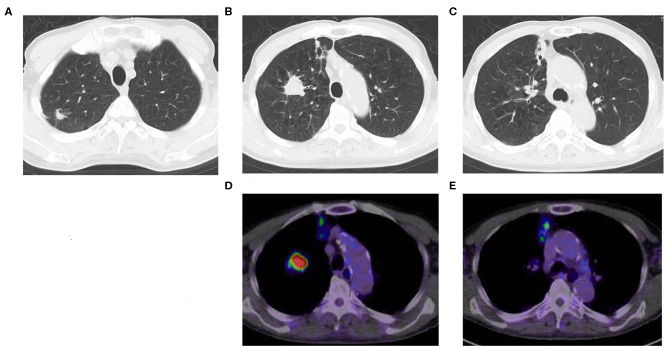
Chest CT shows multiple lung cancers. (A) Adenocarcinoma, (B) pleomorphic carcinoma, (C) adenocarcinoma, (D) positron emission tomography computed tomography (PET-CT) of pleomorphic carcinoma (B), (E) PET-CT of adenocarcinoma (C).
